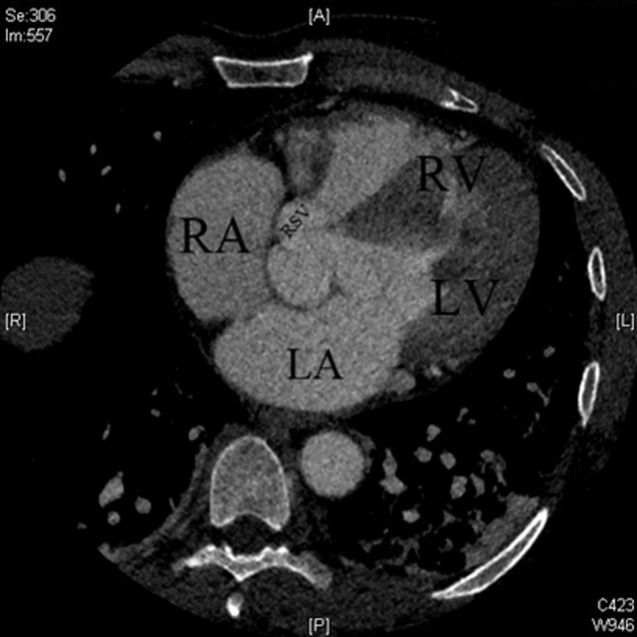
thoracic CT angiography in axial section, showing a passage of contrast material through the right coronary sinus to the right atrioventricular junction
